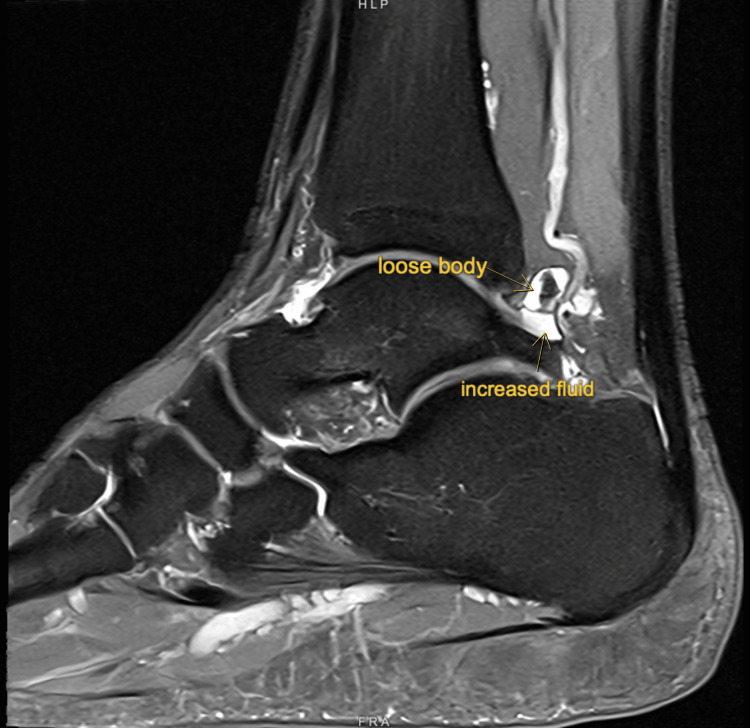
Sagittal MRI of the left ankle demonstrating posterior ankle loose body and increased joint fluid Sagittal fat-suppressed T2-weighted MRI demonstrating a posterior intra-articular loose body (arrow) with associated increased joint fluid, consistent with posterior ankle impingement.
